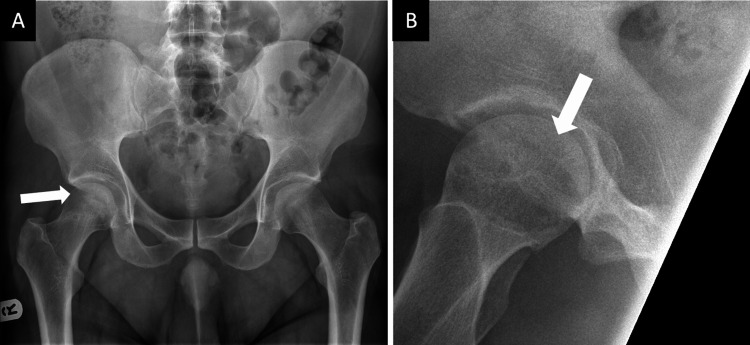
Anteroposterior (A) and lateral (B) pelvic radiographs of the right hip joint demonstrating avascular necrotic lesions (white arrows) in the right femoral head.
